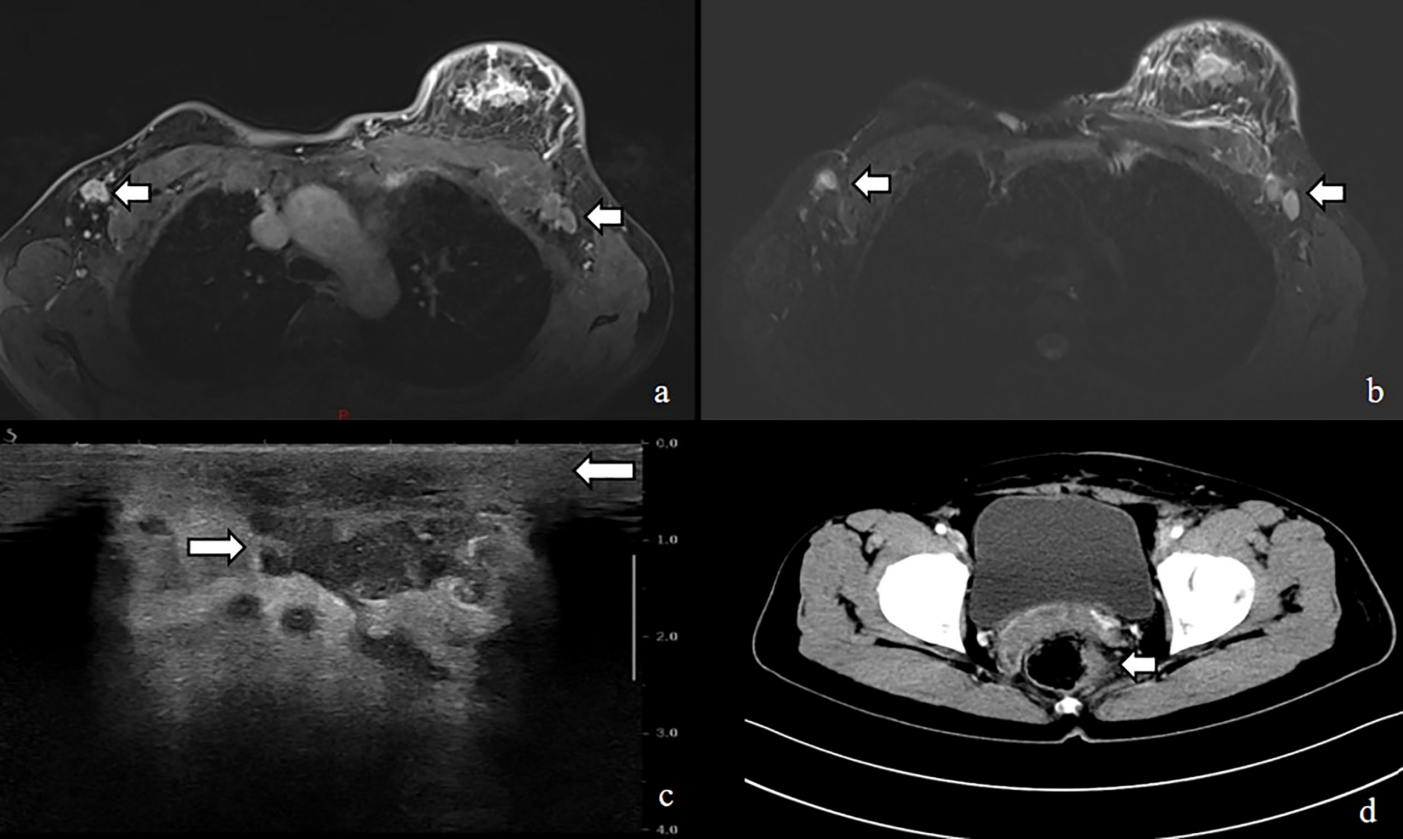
MRI image shows (A, B) enlarged left breast with multiple foci of scattered abnormal enhancement and enlarged lymph nodes in the left axilla, Ultrasound shows (C) thickened subcutaneous soft tissue in the left breast and multiple irregular hypoechoic areas, CT shows (D) uneven thickening and enhancement of the rectal anastomosis wall with small nodules in the adjacent peri-intestinal space.
